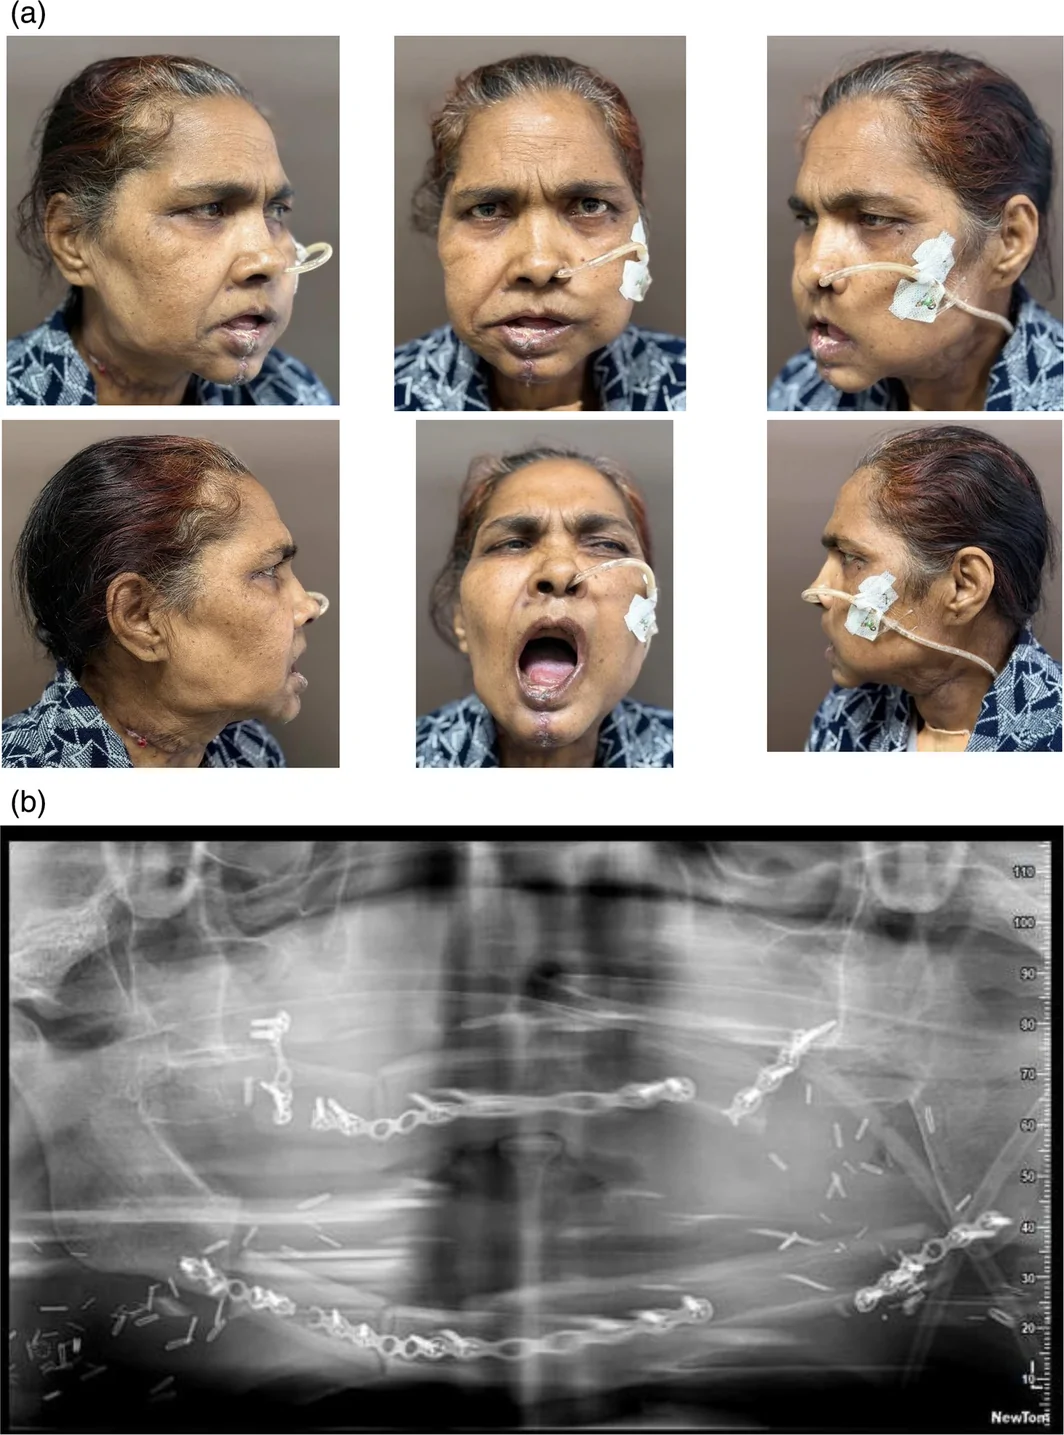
(a) Postoperative picture of the patient on follow up. (b) Postoperative orthopantomogram (OPG) of the patient.
